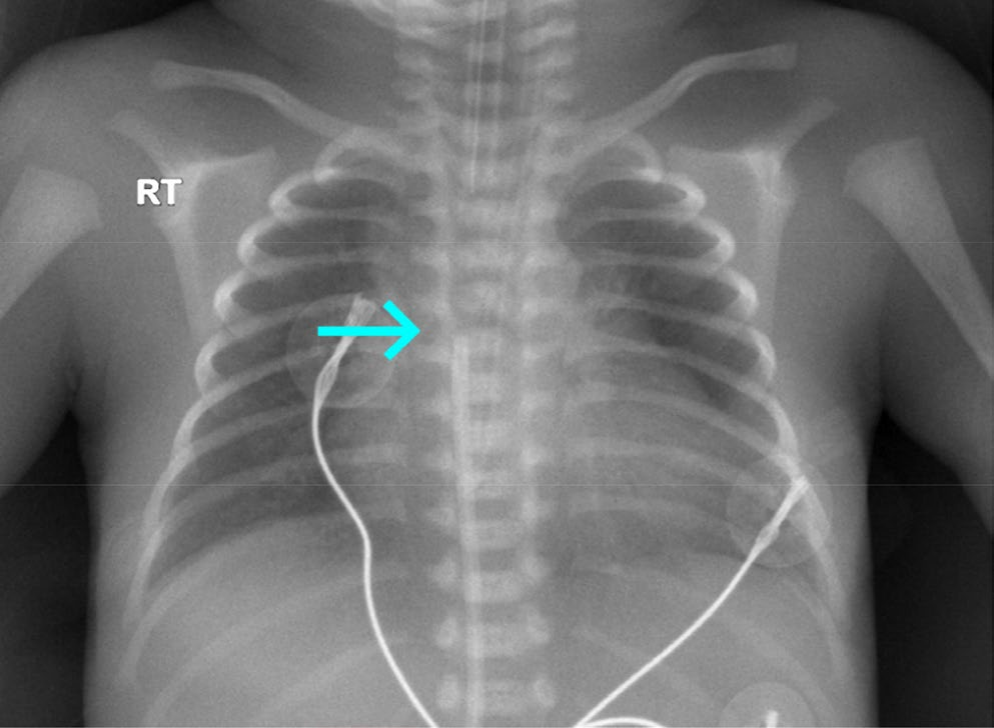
Chest radiography shows the tip of the umbilical venous catheter in the right atrium (displaced)
